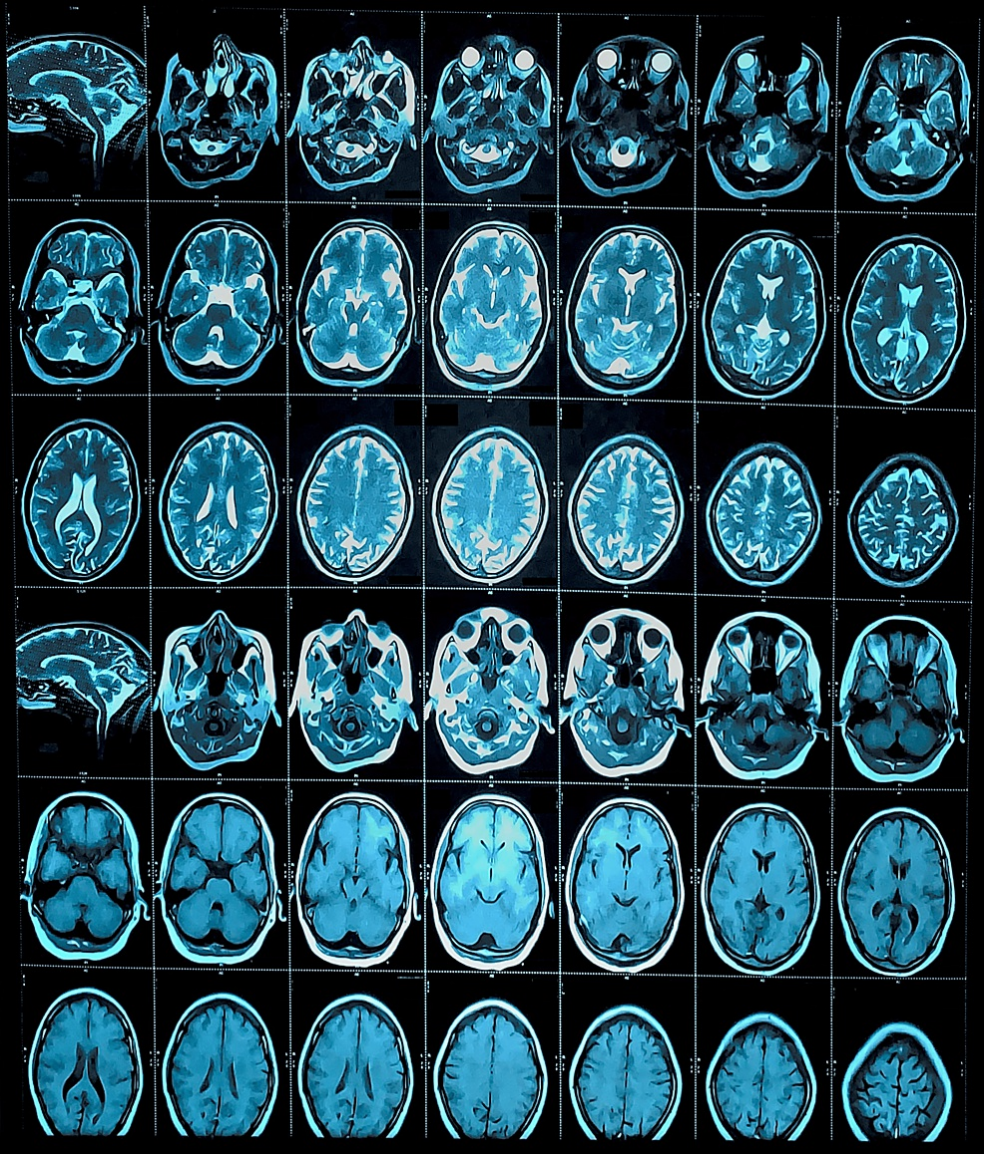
T2 Weighted Axial View MRI of the Brain
